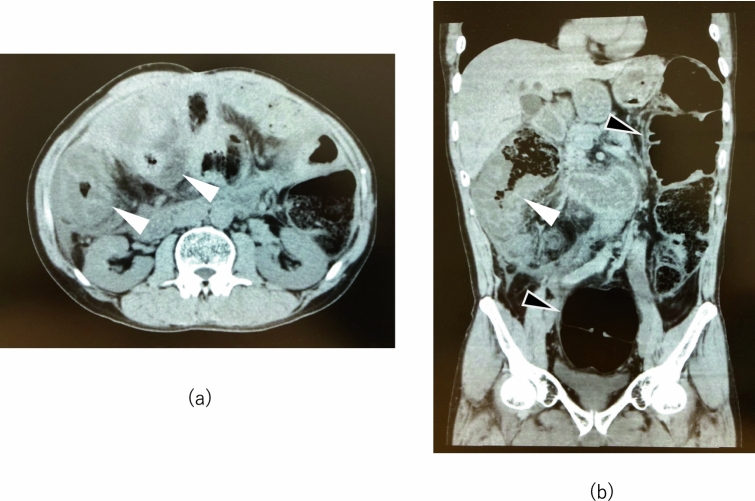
Abdominal computed tomography scan in this HAE-C1-INH patient during an acute attack: a axial section and b coronal section. Submucosal edema of the intestinal tracts is observed during an acute attack in a an axial section and b a coronal section (white arrows). Colectasia is observed in b a coronal section, which was probably caused by the attenuation of peristalsis due to 11 abdominal surgical procedures (black arrows). HAE-C1-INH, hereditary angioedema due to C1-inhibitor deficiency
